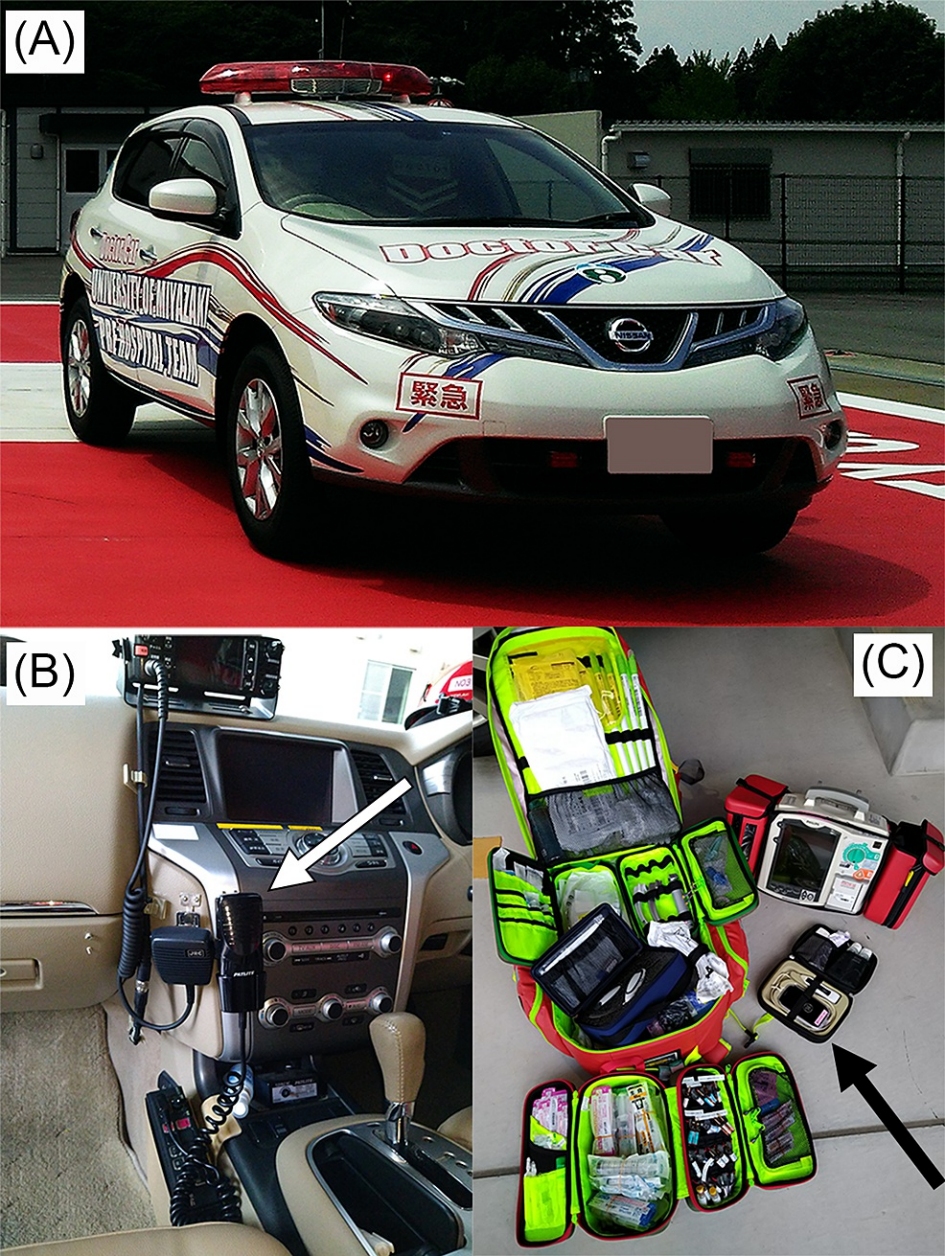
Physician-staffed vehicle (A), view from the seat of the chief physician (B), and medical equipment within the vehicle (C) (A) This vehicle is based on the Nissan Murano® and has been modified as an emergency vehicle. It is equipped with warning lights in front and on the roof. A siren and loudspeaker are attached to the front of the vehicle. Occupants can alert nearby vehicles via the loudspeaker. (B) The chief physician assists the driver by alerting nearby vehicles using the microphone (white arrow). (C) The vehicle carries medical equipment and medications to perform medical interventions, including administration of medication, surgical airway management, tube thoracotomy, resuscitative thoracotomy, pericardiocentesis, manual defibrillation, and percutaneous pacing. The vehicle also carries a portable ultrasound device (black arrow) for emergency ultrasound examination on scene.
